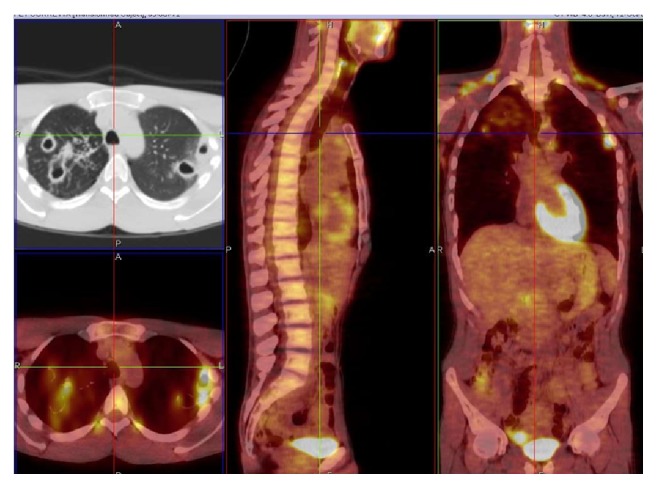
M. kansasii* infection, cavitary lesions in the right upper lobe and in the apical segment of the right lower lobe and left upper lobe, SUV max 5.36. Related to patient 3 (PET/CT. Courtesy “V. Monaldi” Hospital).
